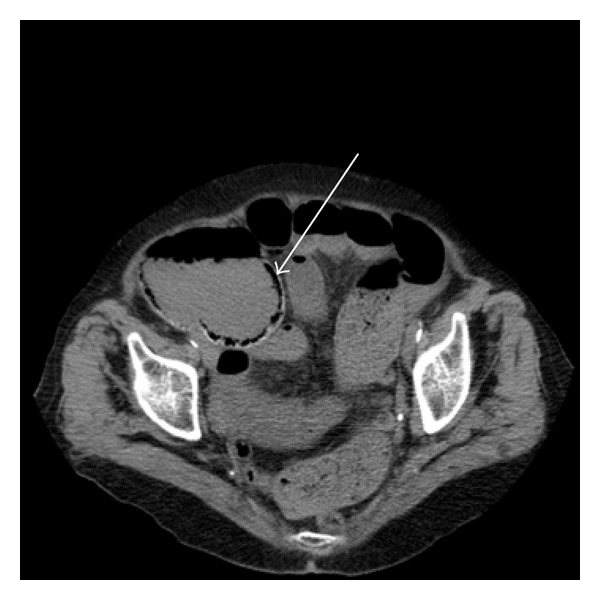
Abdominal CT scan showing air entrapment in the right colonic wall (marked by arrow), colonic liquid levels resembling colonic obstruction, and suspicion of sigmoidal volvulus.
